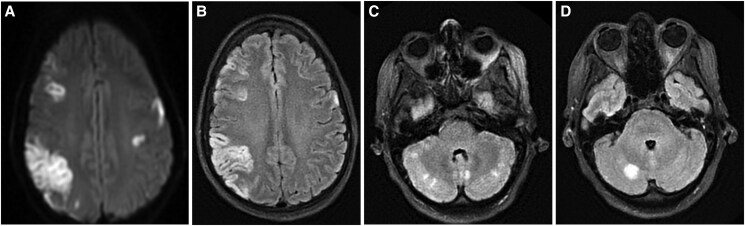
Brain magnetic resonance imaging. (*A*) Diffusion-weighted imaging and (*B*) T2 FLAIR (fluid attenuated inversion recovery) sequences showing a large area of cortical infarction in the right parietal lobe and additional infarcts in the frontal lobes. (*C*) and (*D*) showing acute cerebellar infarcts.
